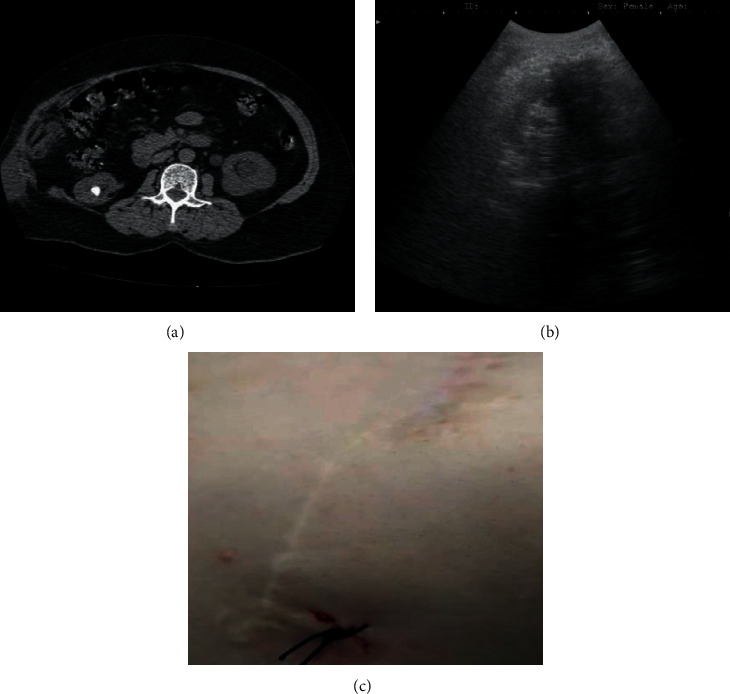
A 54-year-old woman with history of mesh-repaired right incisional flank hernia with right inferior calyx stone and left hydronephrosis; (a) CT scanning view, (b) ultrasound view of hypoechoic shadow of mesh, and (c) surgical hole of PNL and 2 incision lines in flank position for nephrolithotomy and hernia repair.
